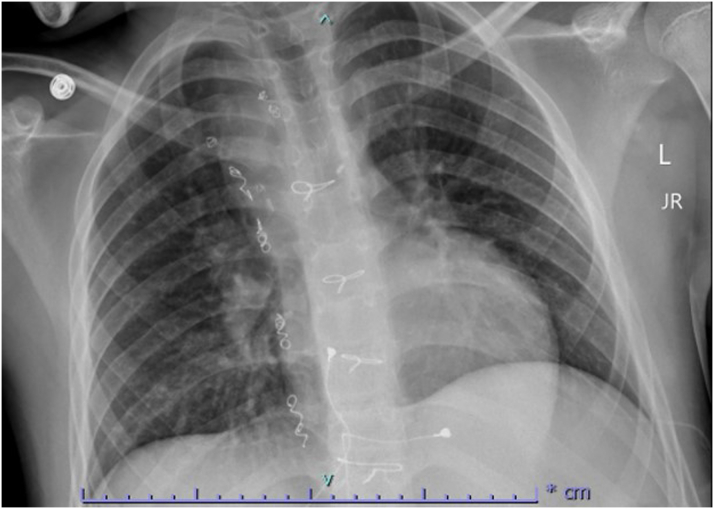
Chest X-Ray at Presentation Chest x-ray with no cardiomegaly, no pulmonary congestion, and with epicardial pacing wires attaching to the atrial and ventricular epicardium and sternal wires from prior sternotomies overlying the central chest.
